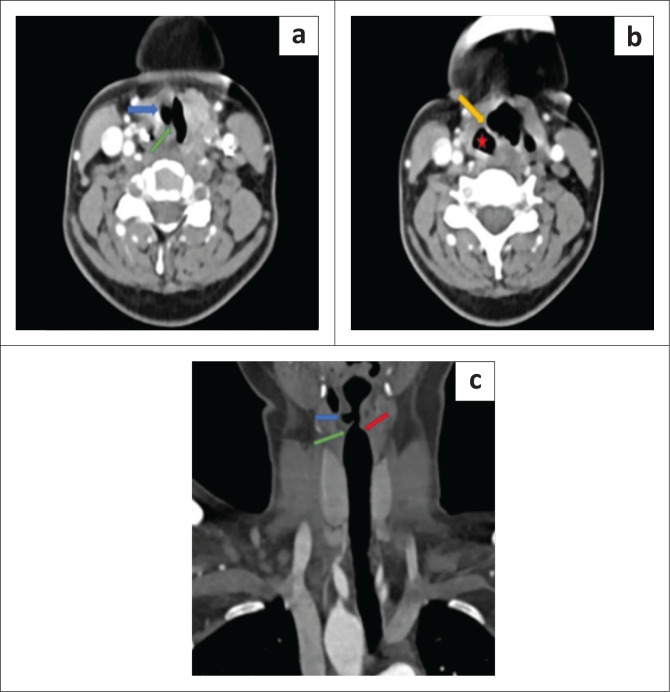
(a, b) Axial contrast-enhanced computed tomography (CECT) neck demonstrates dilatation of the right laryngeal ventricle (blue arrow), relative medial position of the right vocal cord (VC) (green arrow), dilatation of the right pyriform sinus (red star) and medial rotation of the thickened right aryepiglottic fold (yellow arrow), consistent with right vocal cord palsy. (c) Reformatted coronal CECT neck shows dilatation of the right laryngeal ventricle (blue arrow), increased concavity of the angle between the undersurface of the right VC and the right lateral wall of the larynx representing loss of the right subglottic arch (green arrow). Normal subglottic arch on the left side (red arrow).
